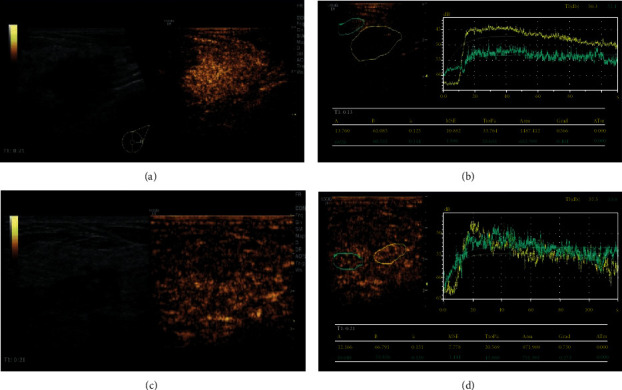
Contrast-enhanced ultrasound before and after NAC in nonluminal breast cancer patients. (a) Contrast-enhanced ultrasound image before NAC showing high enhancement. (b) Time-intensity curve before NAC showing fast-in and high-enhancement relative to adjacent cancer tissue. (c) Contrast-enhanced ultrasound image after NAC. The lesions shrank, showing slightly lower enhancement without enlargement. (d) Time-intensity curve after NAC, showing equal-in and the same enhancement as the adjacent tissues.
